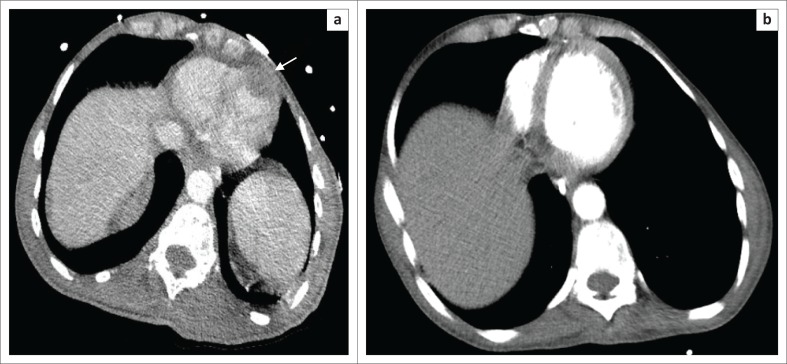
(a) Axial post-contrast thoracic CT imaging demonstrates pericardial tumour infiltration (arrow) with full resolution on (b) post-contrast imaging obtained 1 month later.
